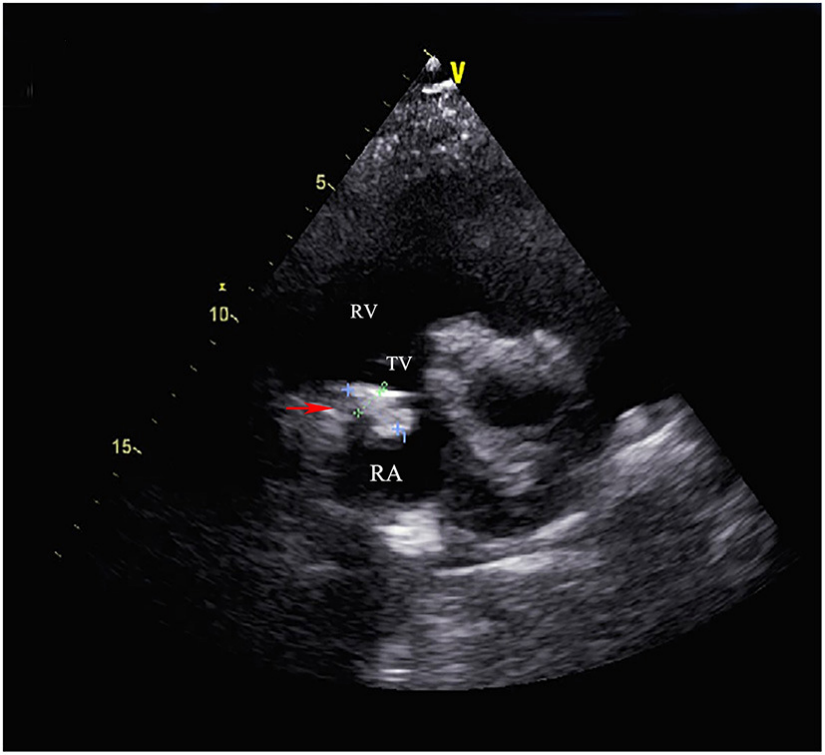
Transthoracic echocardiography showed the cardiac mass (30 mm x 28 mm) located in the right atrium and the anterior tricuspid valve was partially obstructed by the mass but the flow velocity of tricuspid valve did not accelerate. (RA, right atrium; RV, right ventricle; TV, tricuspid valve).
